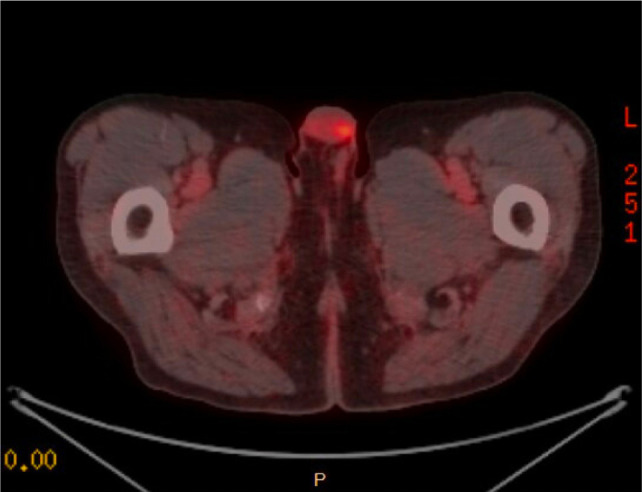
PSMA-PET demonstrating increased uptake in the right testicle, concerning for metastasis.
